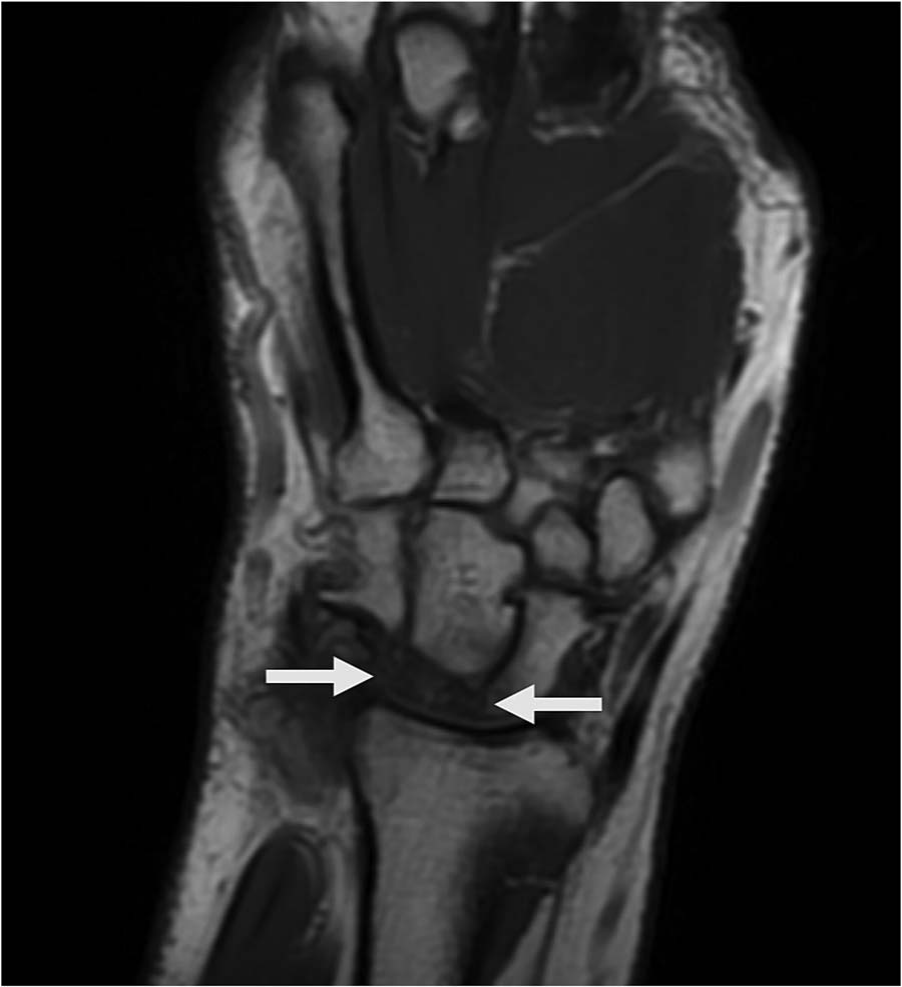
Coronal T1-weighted MR image shows marked diffuse hypointensity of lunate bone and collapse.
